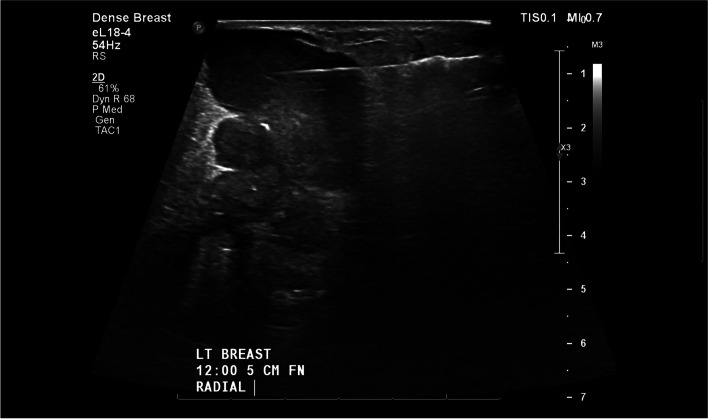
Ultrasound guided core needle biopsy of the irregular heterogenous hypoechoic mass with associated vascularity in left breast at the 12’o’clock position 5 cm from the nipple
